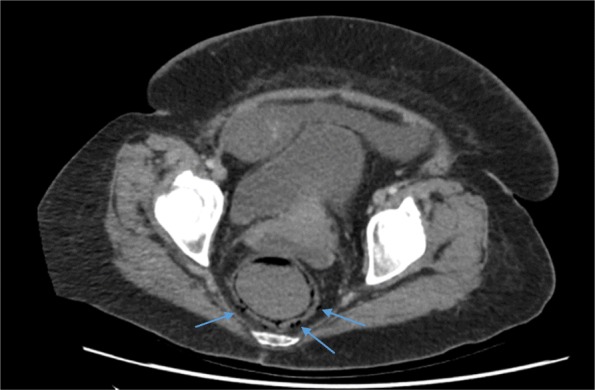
Rectal pneumatosis. Air is present in the wall of the rectum as indicated by *arrows* on coronal section of computed tomography scan of the pelvis
